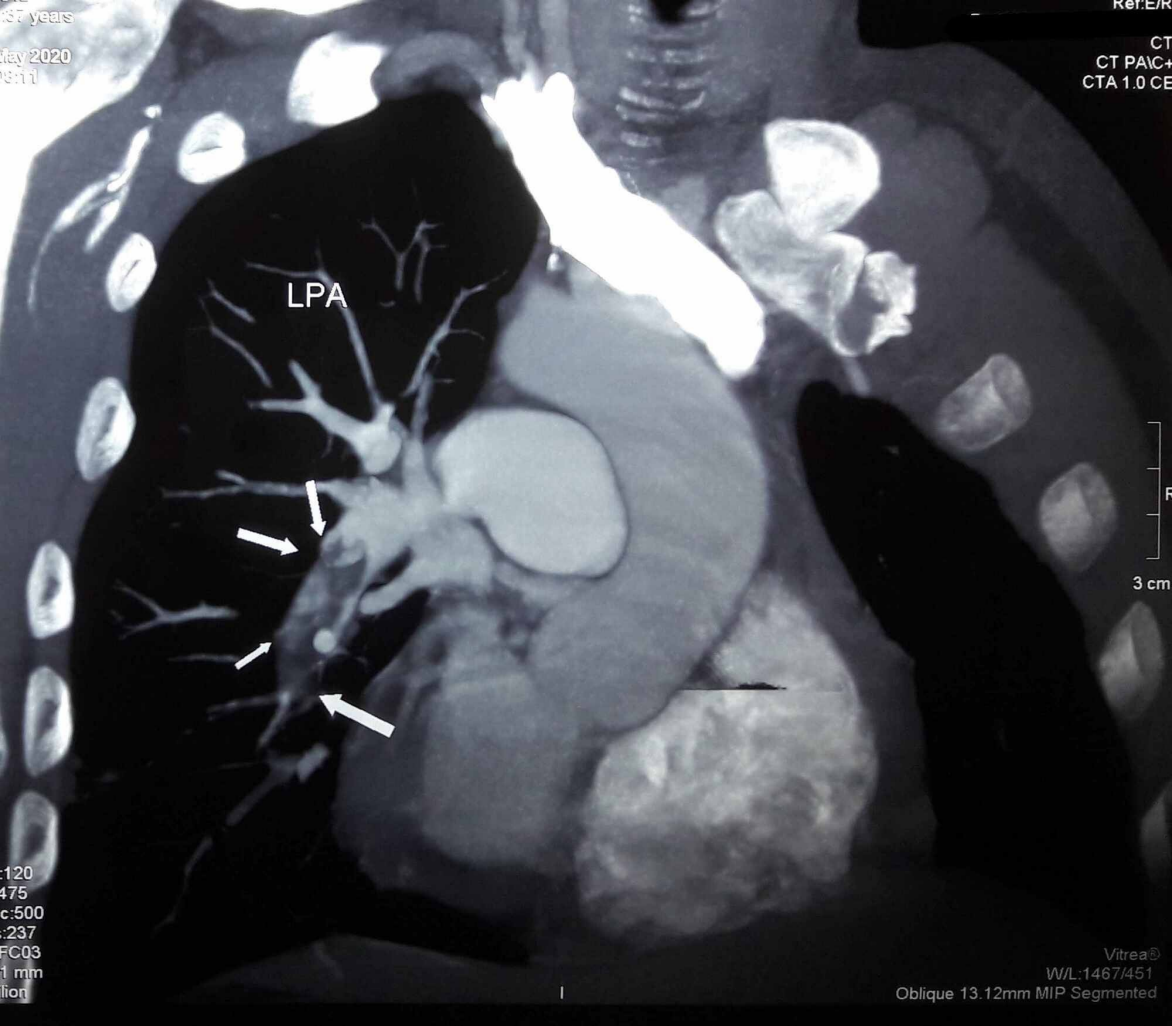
Computed tomographic pulmonary angiography. LPA - left pulmonary artery - occlusive pulmonary thromboembolus involving left pulmonary artery.
